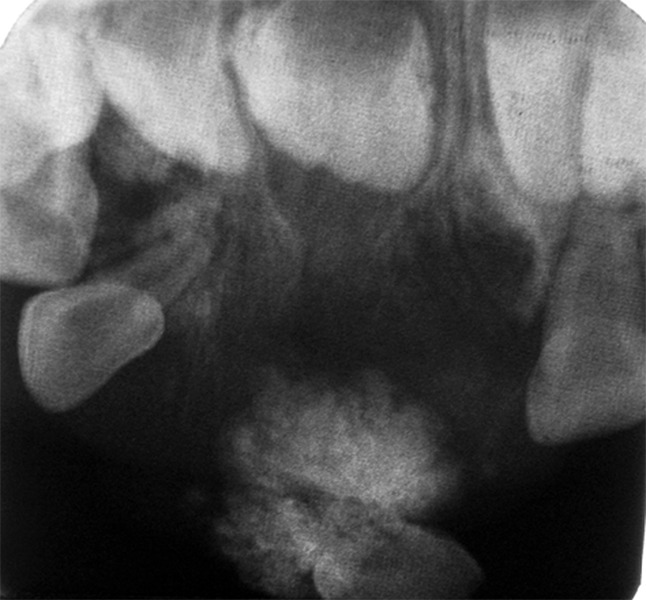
Occlusal radiograph showing mixed type of lesion
